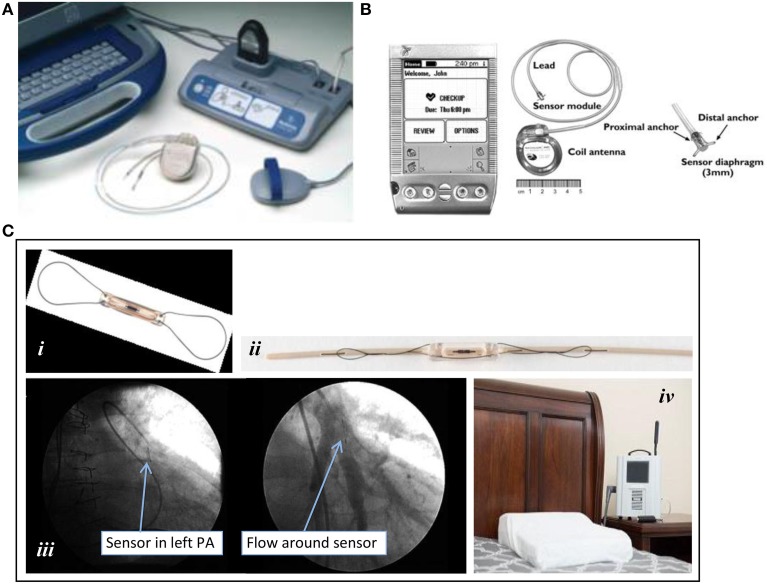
Implantable ambulatory heart failure hemodynamic monitors. (A) Chronicle®: (top left to bottom right) Implantable RV lead and ICD; lateral and posterior-anterior chest radiographs after device placement. (B) HeartPOD®. Implantable left atrial device and distal anchor. (C) CardioMEMS™ HF System: (i) pulmonary artery (PA) sensor; (ii) delivery catheter with preloaded PA sensor; (iii) pulmonary arteriograms showing a radiopaque PA sensor in a segmental branch of the left pulmonary artery (PA) before (left) and after (right) contrast dye injection; (iv) patient electronics system for transmission of data. All images were adapted with permission from Medtronic, Inc., St. Jude Medical, Inc., and Elsevier Inc.
